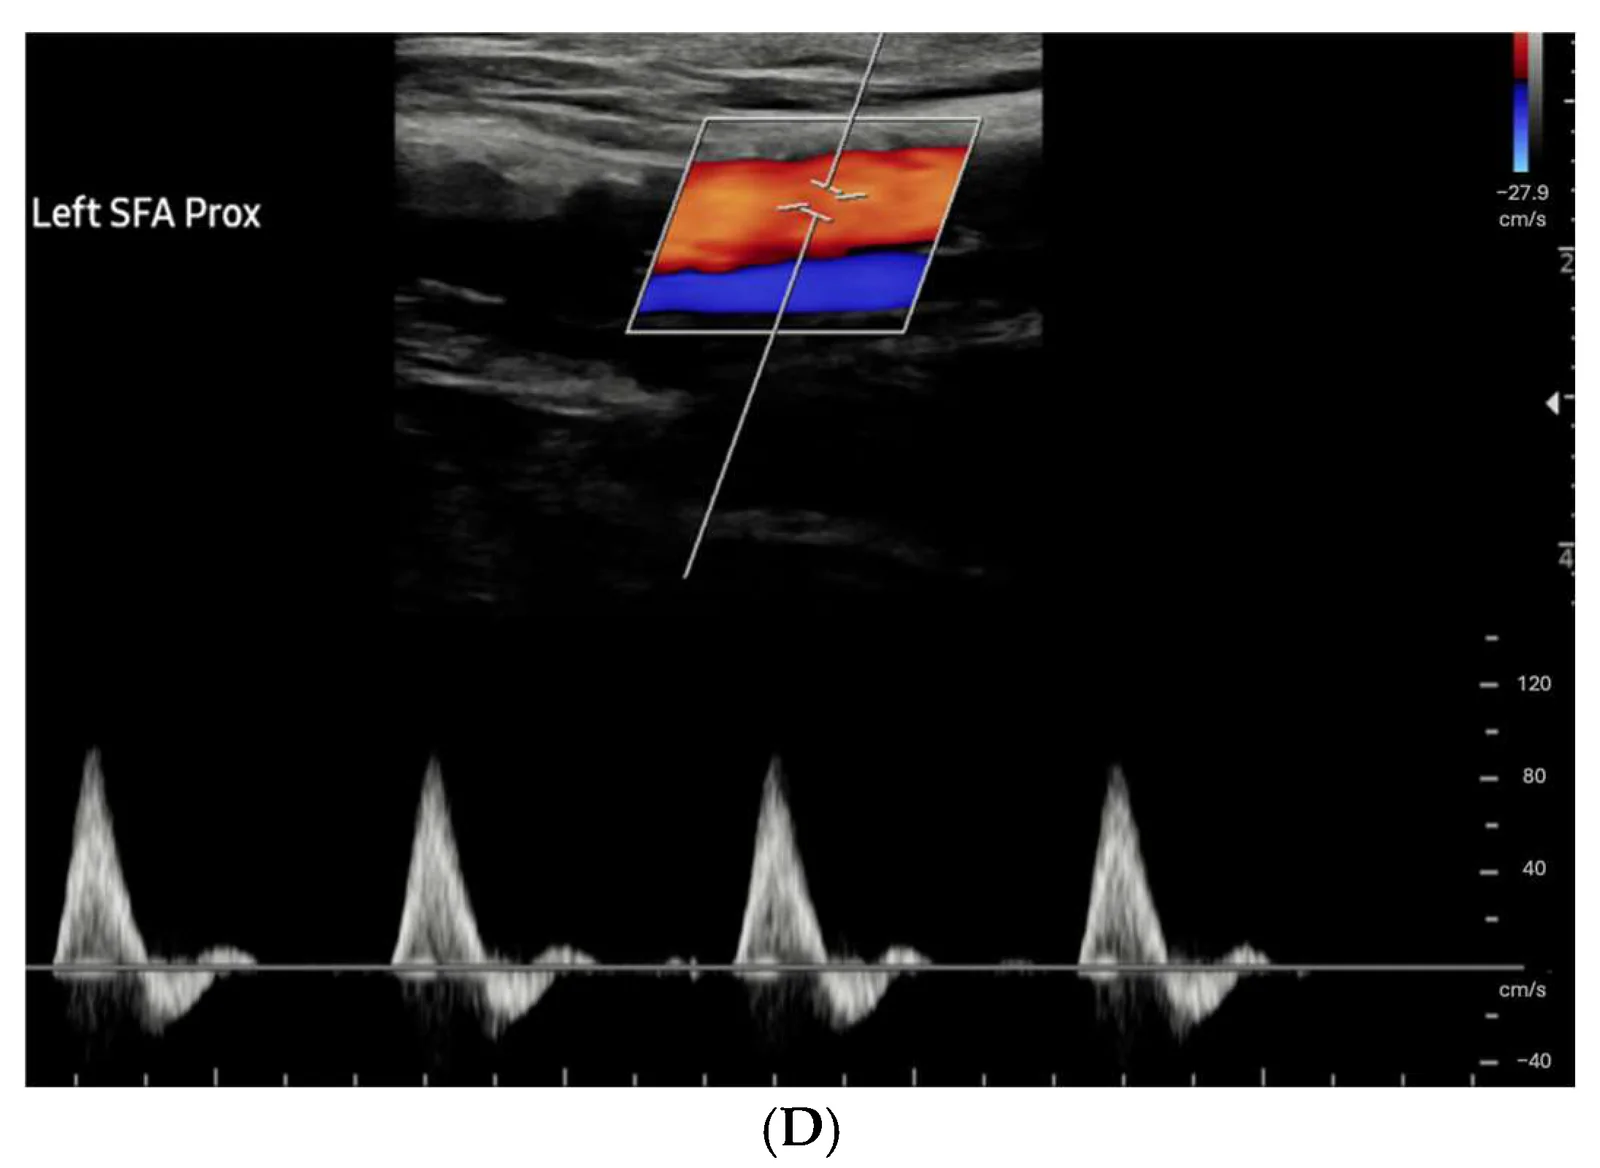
(A) Radiography showing typical “railroad track” pattern of the right common femoral artery (arrow). (B) Radiography showing typical “railroad track” pattern of the popliteal artery (arrow). (C) B-mode ultrasound of the left superficial femoral artery revealing confluent hyperechogenic medial calcification. (D) Doppler ultrasound of the left superficial femoral artery, demonstrating normal triphasic flow spectra.
